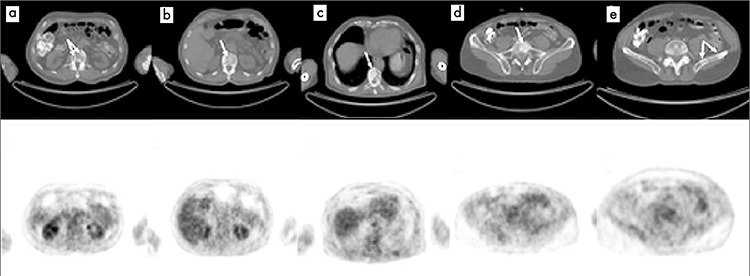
Selected axial slices of PET and CT images from F 18 FDG PET/CT scan obtained after the patient received chemotherapy showing osteblastic lesions at dorsal vertebrae (a, b, c), fifth lumber vertebra (d) and left iliac wing (e) on CT images without FDG avidity
